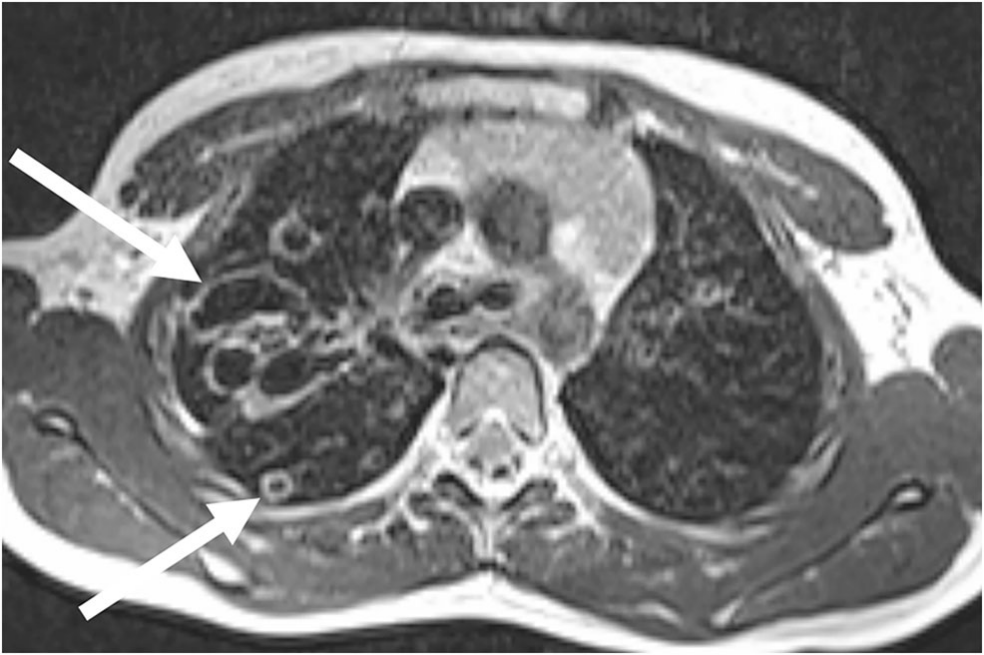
Pulmonary papillomatosis on lung MR in a 5-year-old girl. Axial T2-weighted turbo spin-echo image (MR parameters in Table 2) shows the typical manifestation of pulmonary papillomatosis, with round nodules that are solid or cystic and have a wall of varying thickness (*arrows*). They can grow to several centimeters and then lead to air-filled cavities, with significant destruction of the parenchyma
